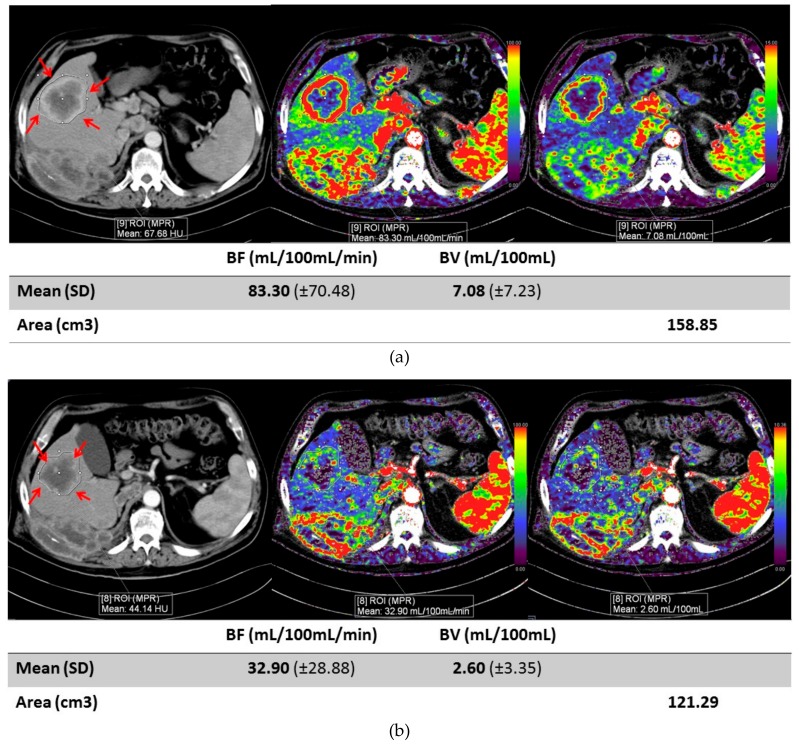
Images of a metastatic lesion. (a) Axial contrast enhanced image and CT perfusion images before therapy in patient with renal cell carcinoma metastases to the liver (red arrows). Blood flow is 83.3 mL/100 mL/min and blood volume is 7.08 mL/100 mL. (b) Axial contrast enhanced image and CT perfusion image of same patient eight days after therapy shows dramatic decrease in blood flow to 32.90 mL/100 mL/min and blood volume decrease to 3.35 mL/100 mL. Standard deviation (SD) for each measurement is shown on the table below the figure.
